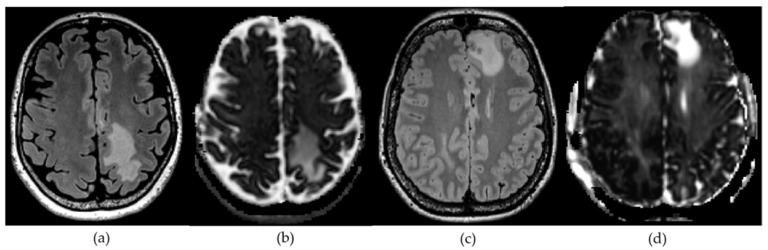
Axial FLAIR (a,c) and parametric V-CSF-maps (b,d) in a left centroparietal GBM (a,b) and left frontal metastasis (c,d). Of note, the metastasis shows a relative increase in perilesional V-CSF compared with GBM (d,b).
